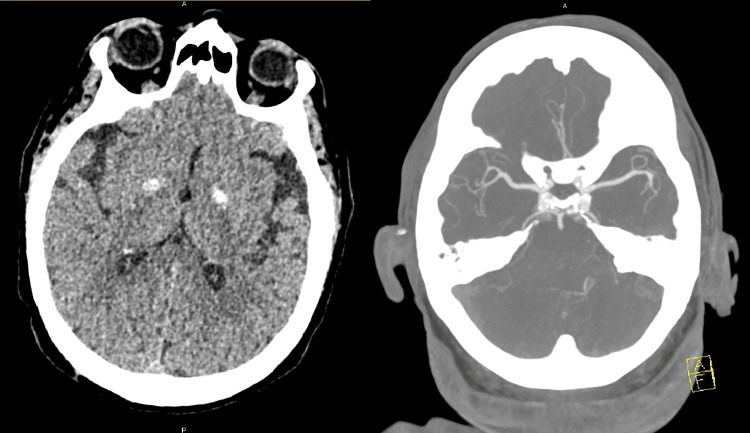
Non-contrast head CT and CT angiography findings Non-contrast head CT demonstrating bilateral basal ganglia dystrophic calcifications and absence of ischemic changes (left), and CT angiography demonstrating no large vessel occlusion (right) CT: computed tomography
